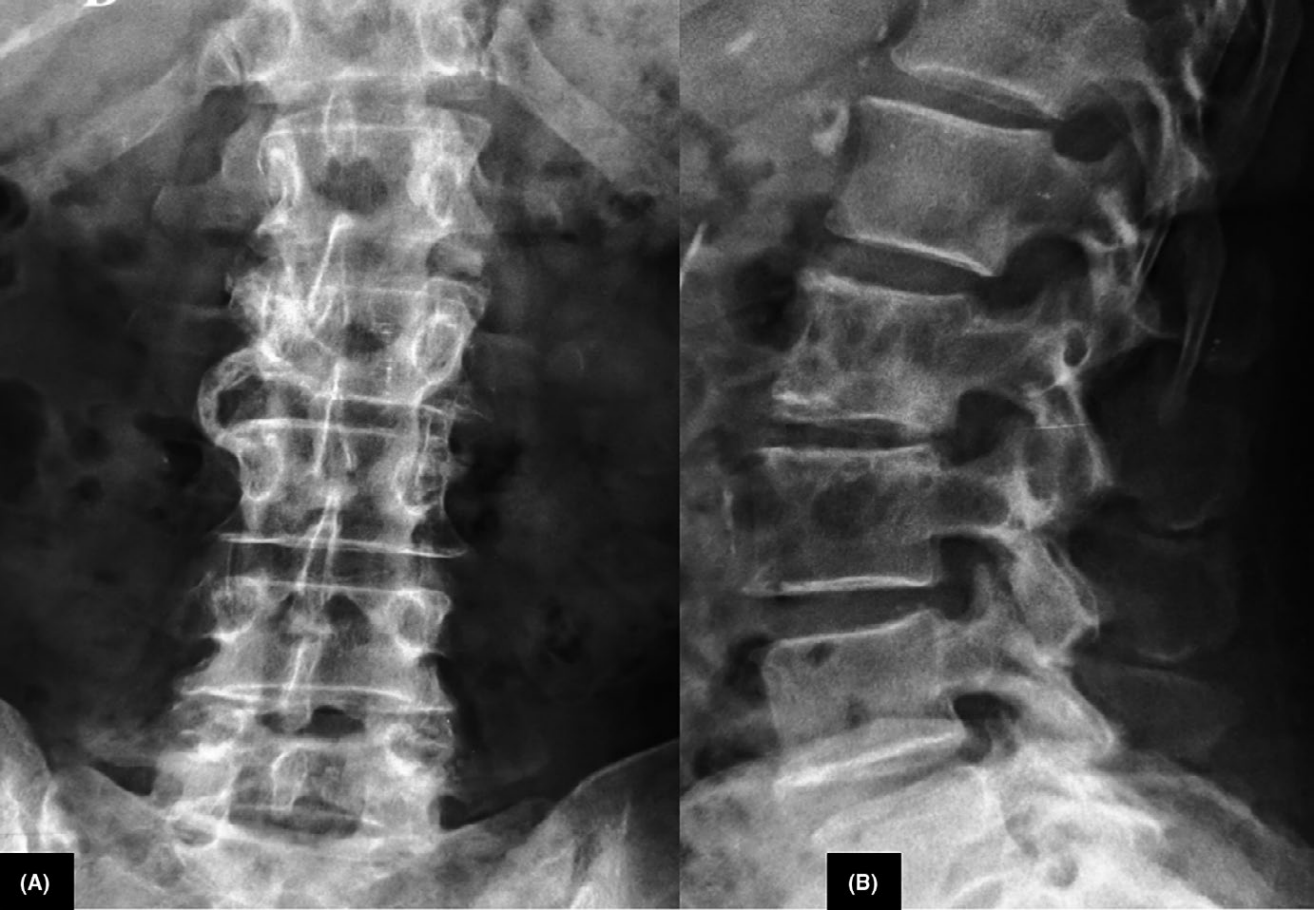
Anteroposterior (A) and lateral (B) radiographs of lumbar spine: mixed lytic‐sclerotic pattern in the L2 vertebra
